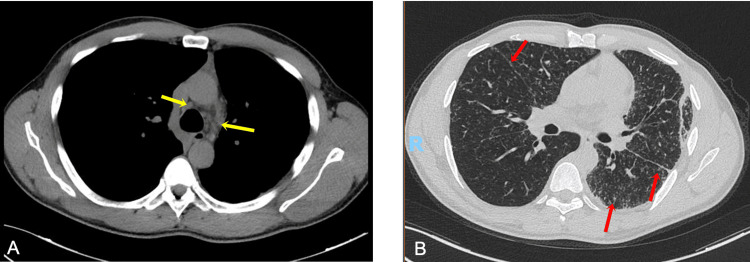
Follow-up chest CT scan after seven months of corticosteroid treatment. Image A shows reduction in the mediastinal lymphadenopathies (yellow arrows). Image B shows improvement of the pleural thickening (yellow arrow) and persistence of the nodular pattern with perilymphatic distribution predominantly in the left lung (red arrows).
